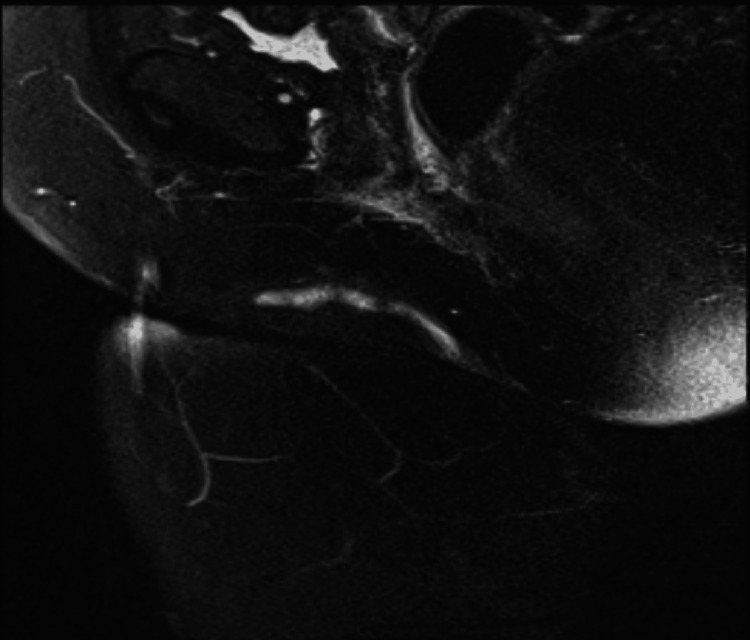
MRI Pelvis showing sinus tract
